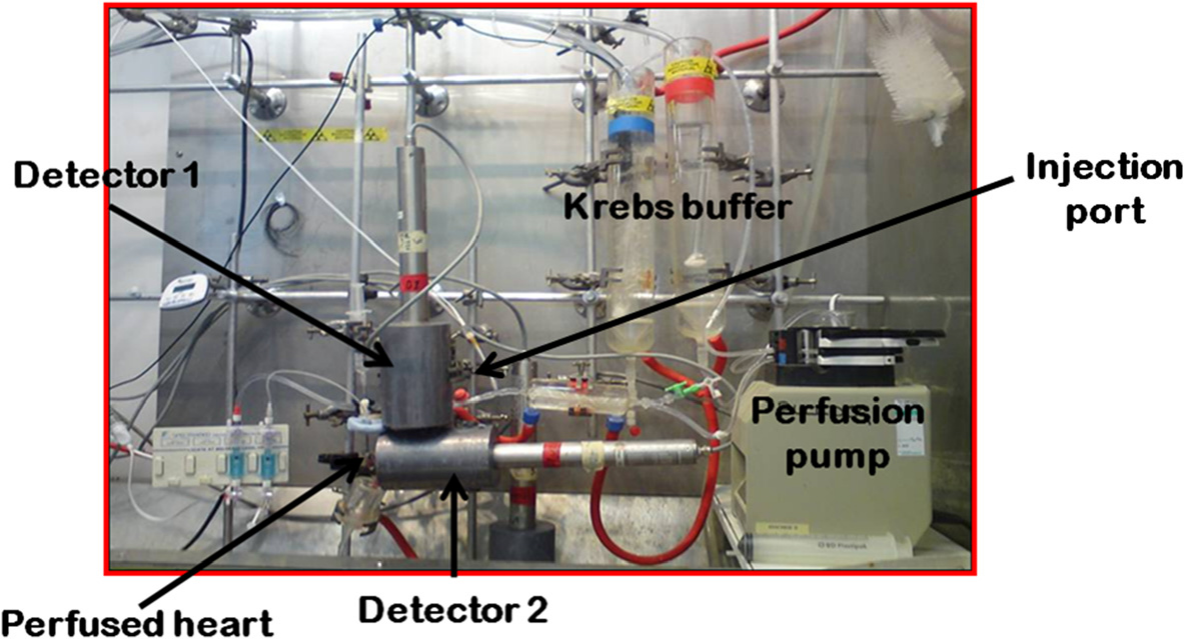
Experimental set-up. In each experiment, a bolus of [^18^ F]-FDG or [^18^ F]-FMISO was injected through the injection port, and its passage through the perfusion line was recorded using two NaI γ-detectors which are able to measure the input (detector 1) and the tissue (detector 2) time-activity curves.
